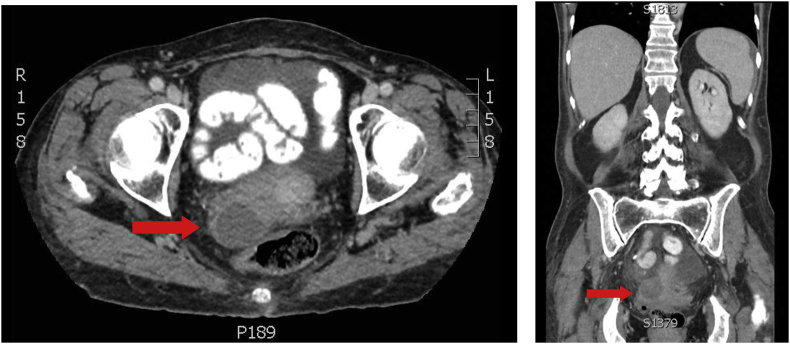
Repeat CT imaging of the abdomen and pelvis four months after surgical revision and abscess reformation shows stable complex fluid collection at 3.2 × 3.0cm from 3.0 × 2.9cm four months prior.
